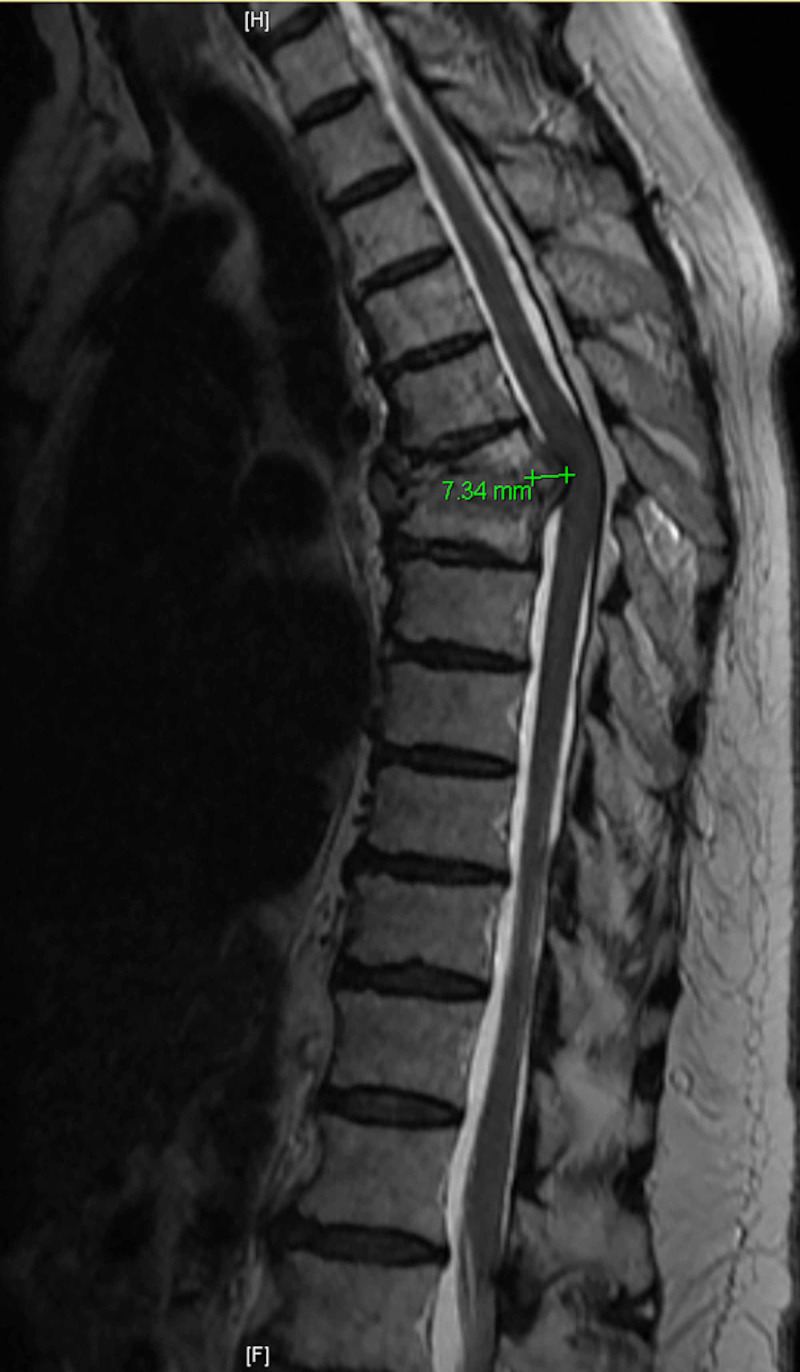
Sagittal T2 MRI of the thoracic spine indicating the presence of a severe kyphotic deformity from T6-T7 due to erosive lesion within the vertebral bodies with associated dorsal mass effect and severe spinal cord compression with signal cord change
